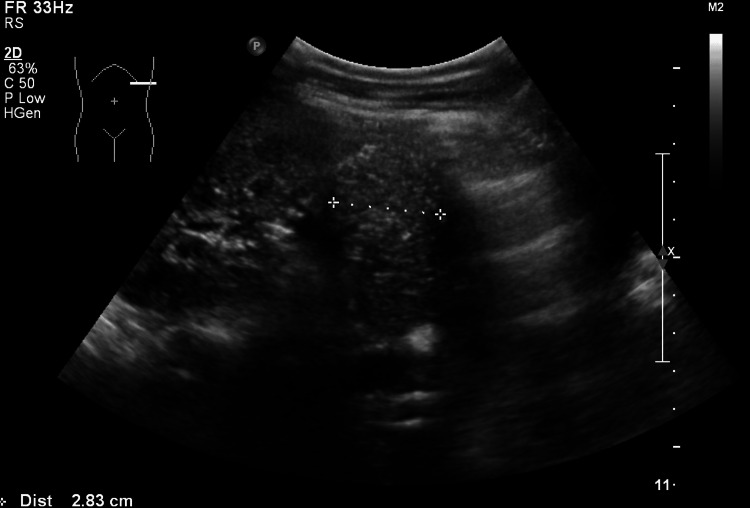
Abdominal ultrasound showing a cyst located within the pancreatic parenchyma.
